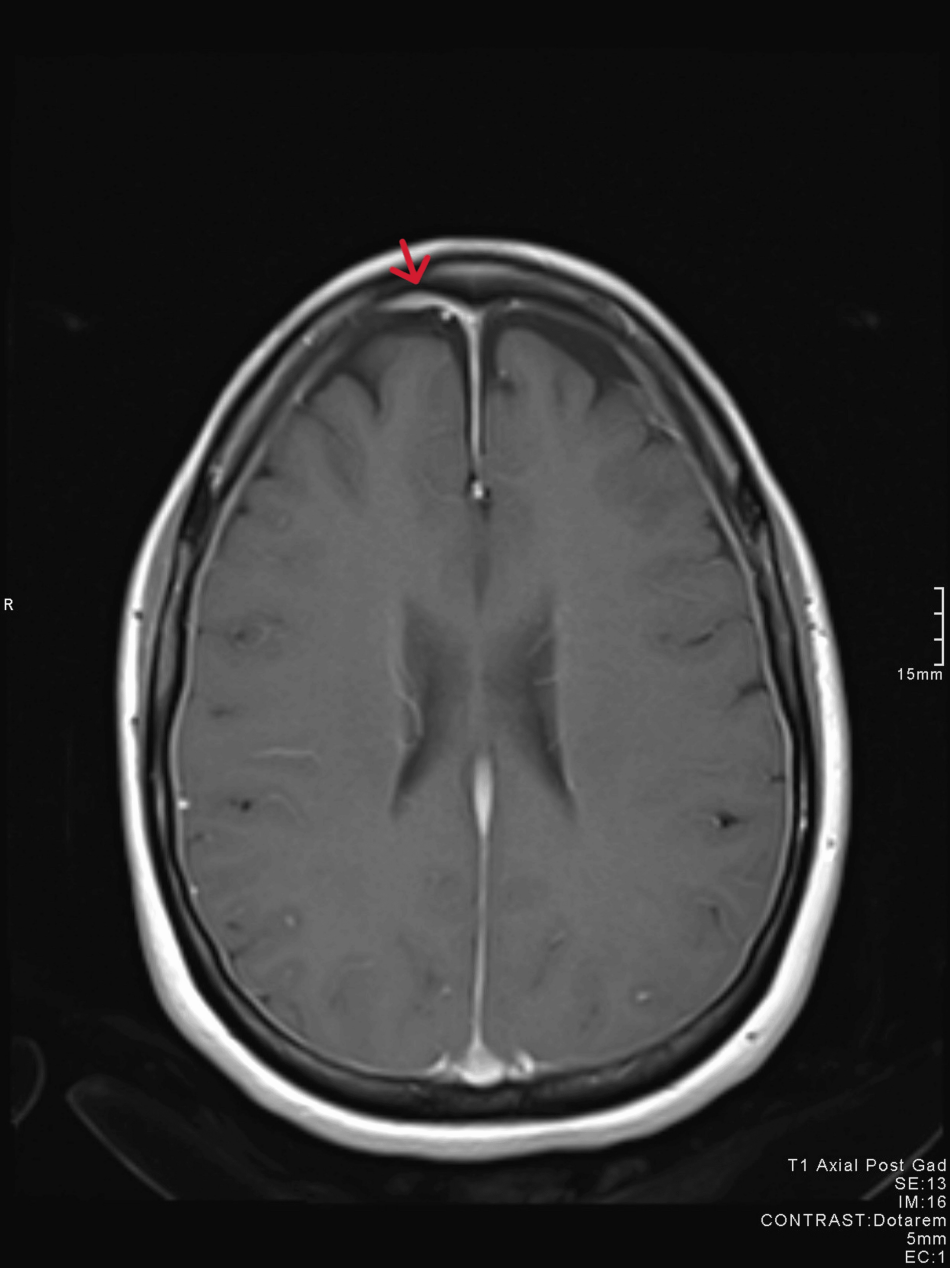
MRI axial view showing pachymeningeal enhancement.
